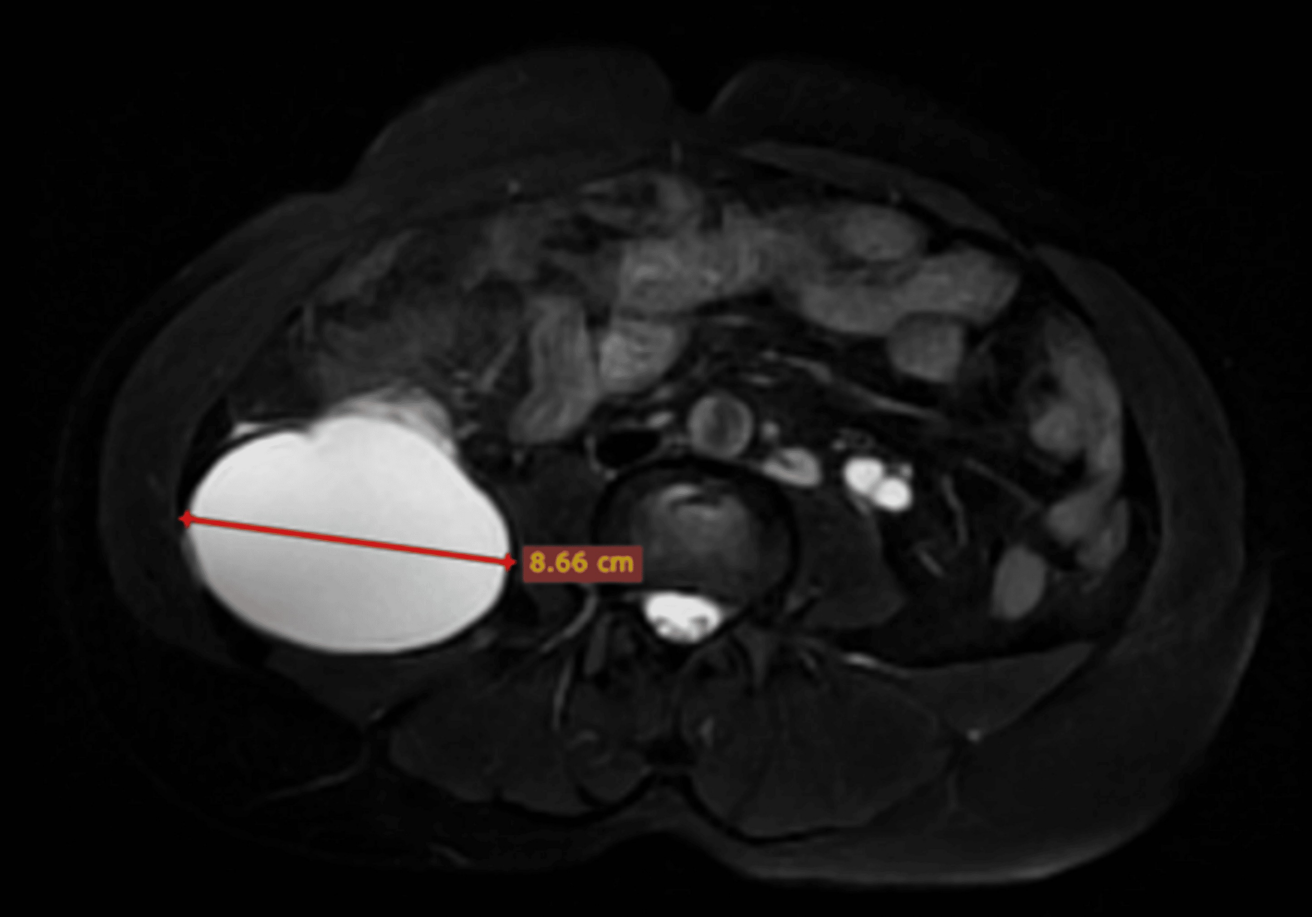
Non-contrast fat-saturated T2-weighted MRI of the abdomen (axial view) showing a hyperintense collection in the right lumbar region measuring 8.6 cm in the maximum transverse dimension (blue arrow).
